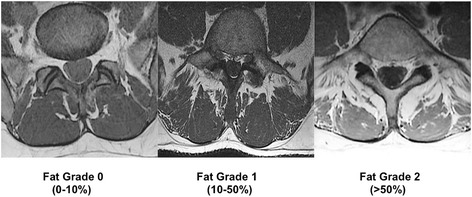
Grading of MR images with different muscle-fat compositions of lumbar multifidus muscle. The slice demonstrating the highest quantity of fat infiltration was graded accordingly
